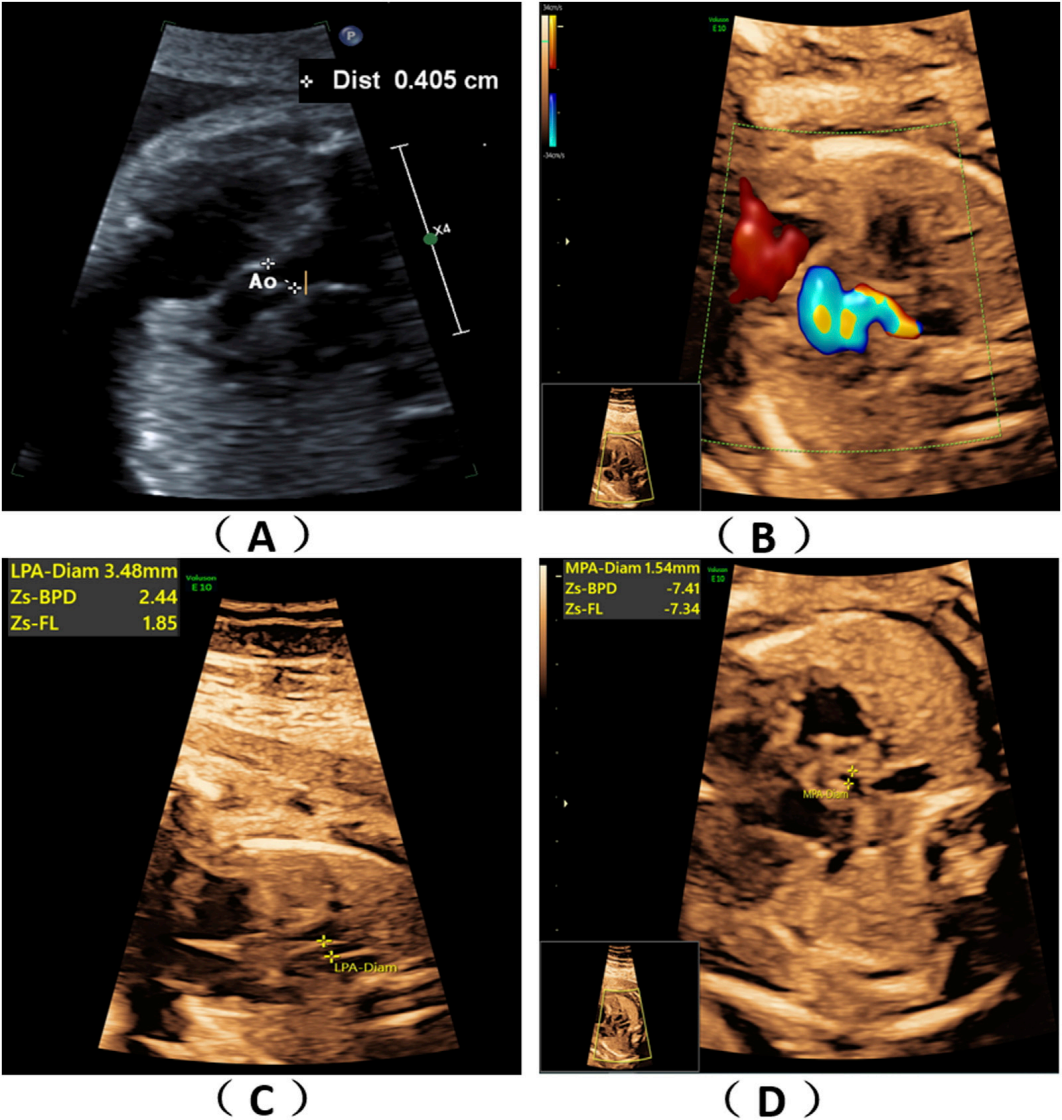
Fetal ultrasound scan findings. Ultrasound scans of the fetus showed fetal congenital heart disease including pulmonary atresia, regurgitation and tricuspid valve dysplasia at the 24th week of gestation (A–D).
